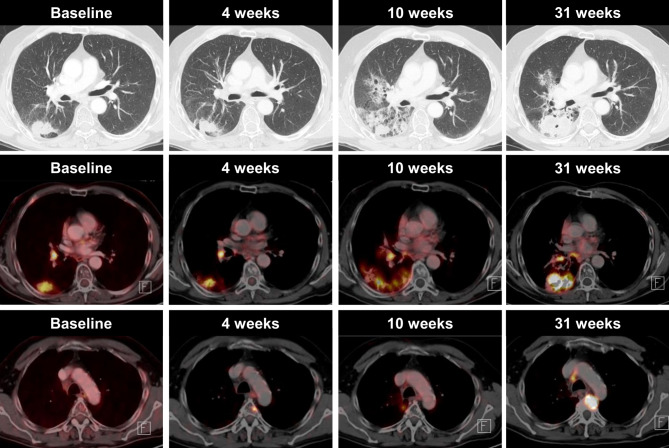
A case of pseudoprogression in a 52-year-old man with NSLCL, who was receiving immune checkpoint inhibitor therapy. Axial CT and ^18^F‑FDG-PET/CT imaging obtained before the start of treatment show a metabolically active tumor in the right lower lobe and an ipsilateral hilar lymph node metastasis. Four weeks after treatment initiation, the tumor slightly decreased in size and showed reduced ^18^F‑FDG uptake. However, we observed a newly developed, metabolically active metastasis in the 4th thoracic vertebral body measuring <1.0 cm. Treatment was continued and PET-CT performed 10 weeks after the start of treatment showed no increased ^18^F‑FDG uptake in the 4th thoracic vertebral body. The ^18^F‑FDG uptake in the primary tumour in the right lower lobe further decreased. The newly developed, metabolically active, ground-glass opacities were radiation-associated. Thirty-one weeks after the start of treatment, ^18^F‑FDG PET-CT evidenced disease progression in both the primary tumour and the metastasis in the 4th thoracic vertebral body. Upper row: CT in lung window, middle row: axial fused PET/CT image lower row: axial fused PET/CT image
